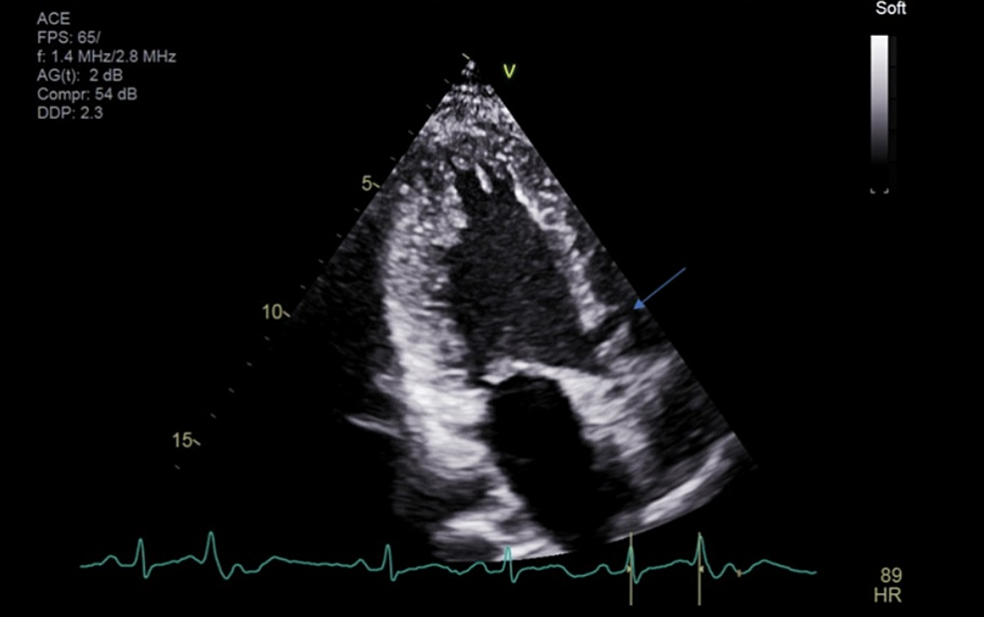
Echocardiogram – left Ventricular ejection fraction 55-60%. Subpulmonic VSD with Left to Right Shunt.
